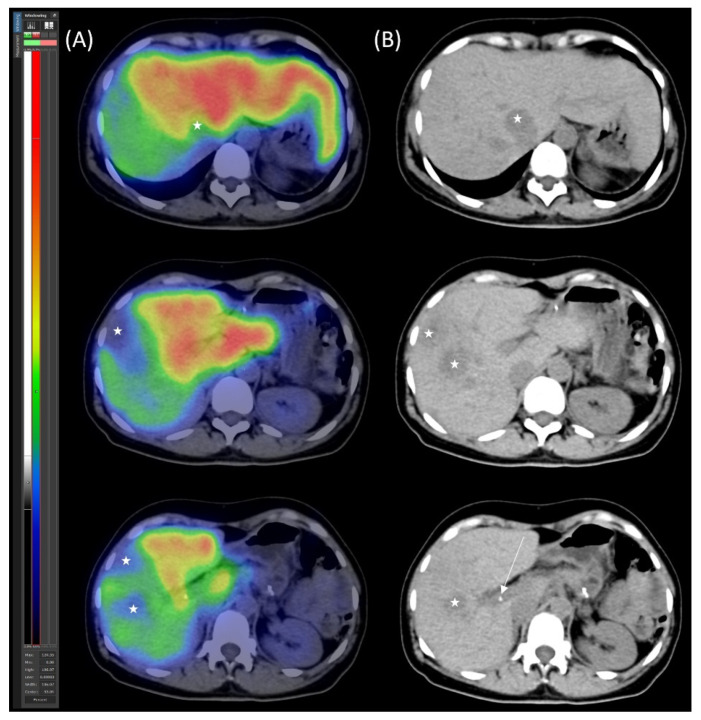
[^99m^Tc]Tc-mebrofenin SPECT/CT after parenchymal modulation. (A) Transaxial SPECT/CT and (B) native low-dose CT images show the distribution of functional hepatocytes, highlighting the dominance of the hypertrophied left lobe. The right lobe metastases appear as photopenic hypodense areas (marked with stars), with the arrow indicating the right portal vein ligation.
